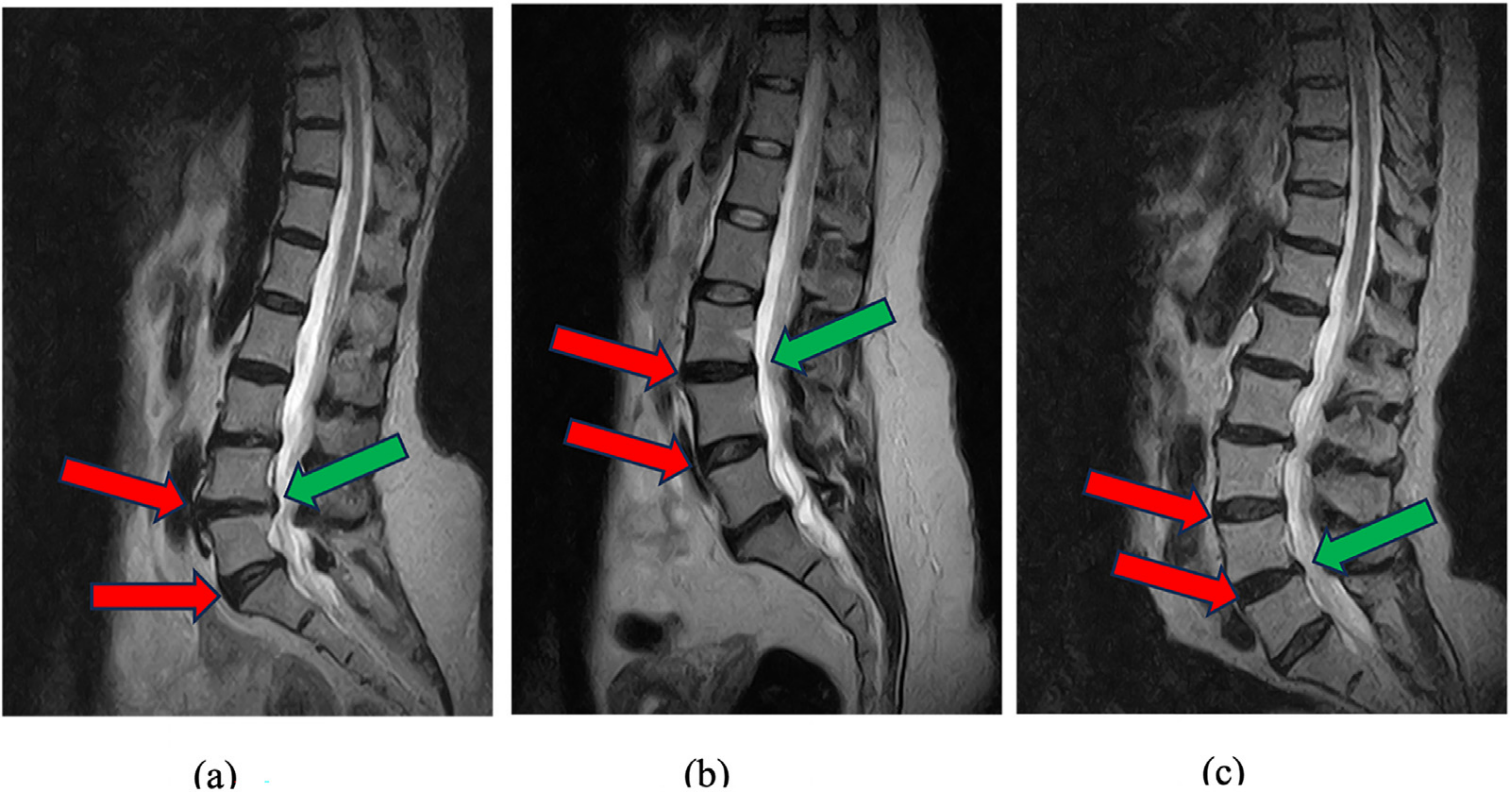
Lumbar T2 magnetic resonance imaging mid-sagittal slices showing cases of disagreement on Spondylolisthesis grade between SpineNetV2 and radiologist. (A), L4-L5 and L5-S1 intervertebral discs graded as grade 1 by SpineNetV2 (red arrow) but only L4-L5 disc is grade 1 by radiologist (green arrow). (B), L3-L4 and L4-L5 intervertebral discs graded as grade 1 by SpineNetV2 (red arrow) but only L3-L4 disc is grade 1 by radiologist (green arrow). (C), L3-L4 and L4-L5 intervertebral discs graded as grade 1 by SpineNetV2 (red arrow) but only L4-L5 is grade 1 by radiologist (green arrow).
